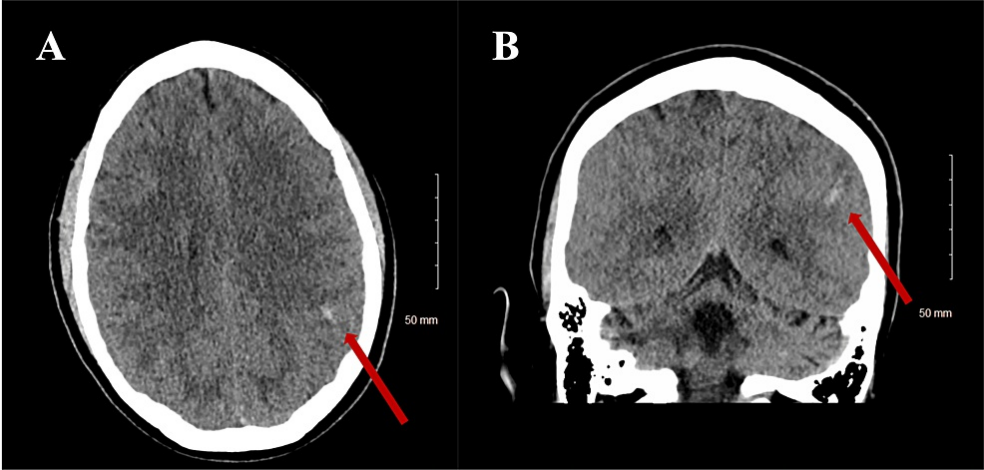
Non-Contrast CT of the Head (A) Axial non-contrast CT head showing one of the two foci of intraparenchymal hemorrhages. (B) Non-contrast CT head showing a small intraparenchymal hemorrhage on the coronal sequence. CT: computed tomography
